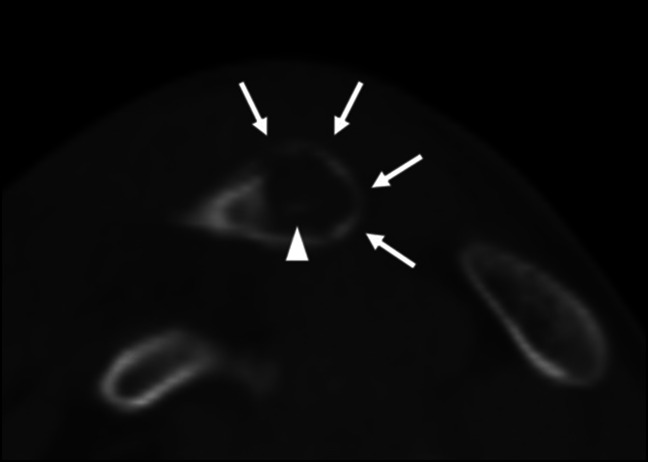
Sagittal CT (2 mm cuts) of the clavicle demonstrating cortical erosion (arrows) with an intramedullary sequestrum (arrowhead).
